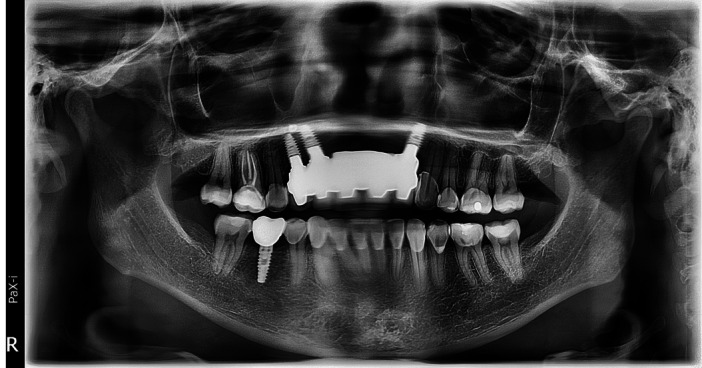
A panoramic radiograph after 36 months of follow‐up, showcasing the surgical repair for the vertical defect and oronasal communication, along with implants with stable bone.
